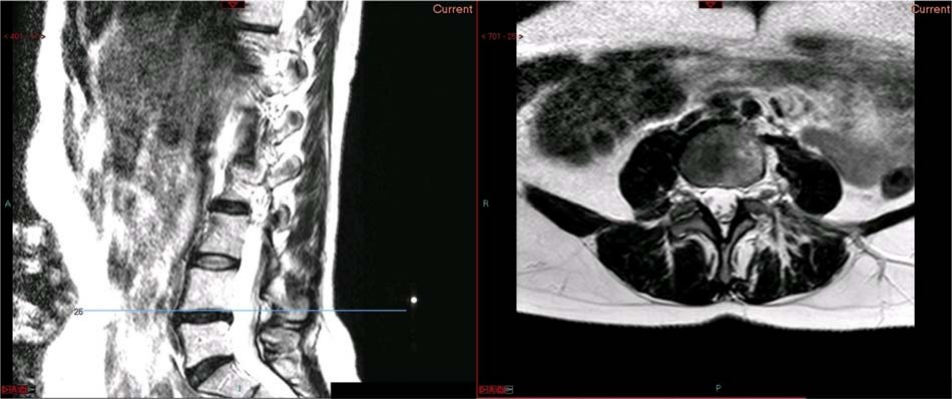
Preoperative MRI scan of the lumbar spine: preoperative MRI scan of the lumbar spine performed in October 2013, showing non-compressive disc degeneration at the L4/5 and L5/S1 vertebrae.
